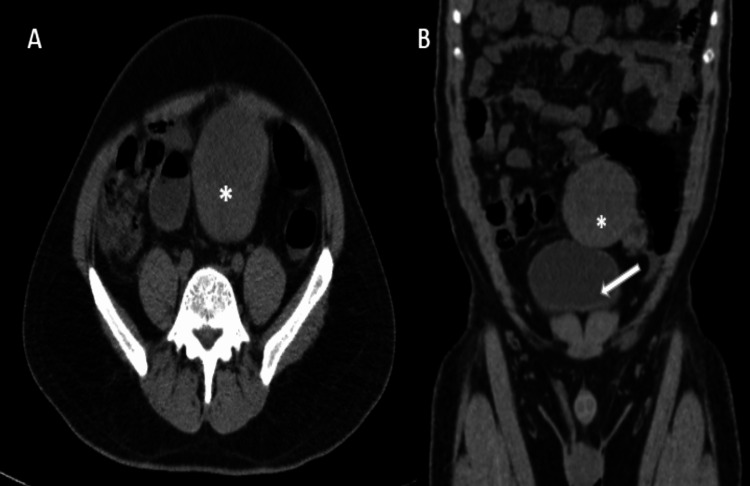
Axial (A) and coronal (B) section of plain CT abdomen showing well-defined lobulated solid soft tissue attenuation mass (asterisk) in the left hypogastric region in the supra-vesical area (urinary bladder marked by white arrow)
